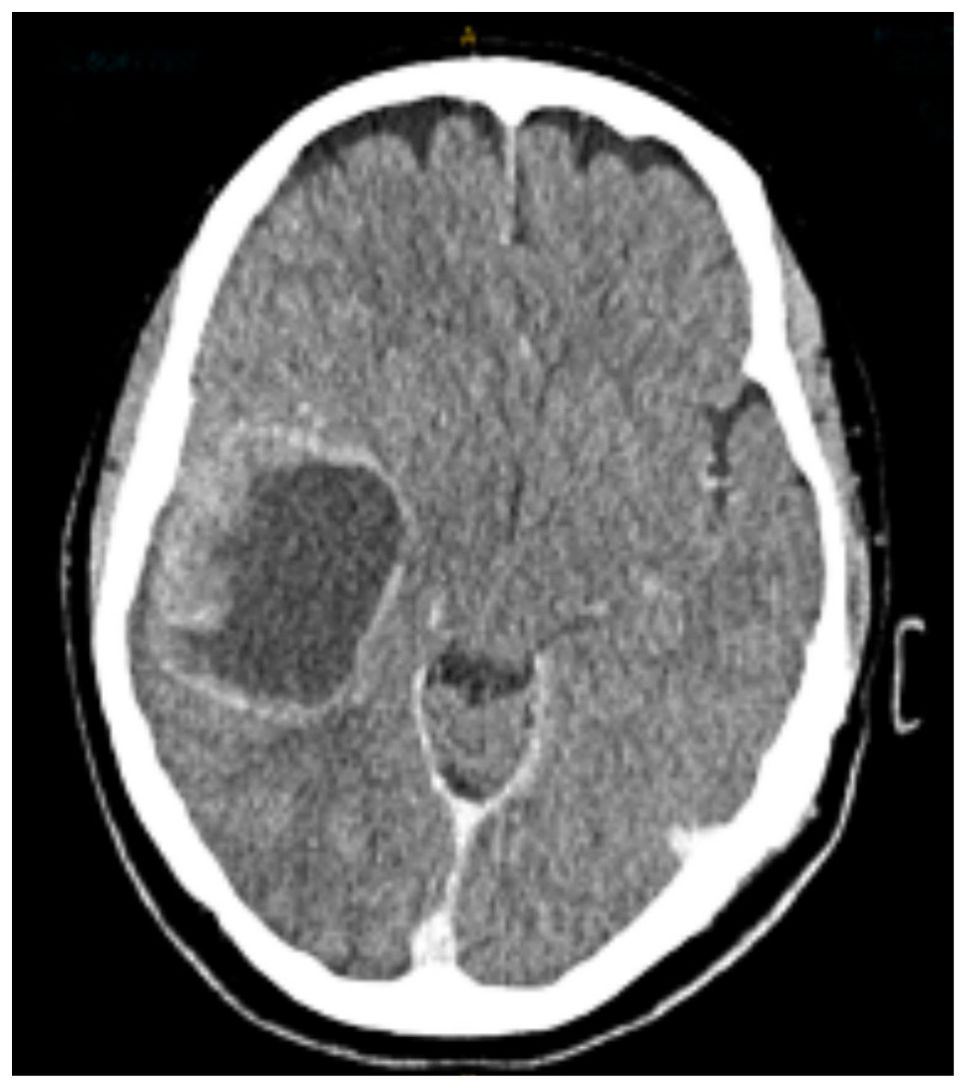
Post-contrast axial CT scan demonstrating large peripherally enhancing heterogenous solid-cystic tumour with localised mass effect. CT, computed tomography.
